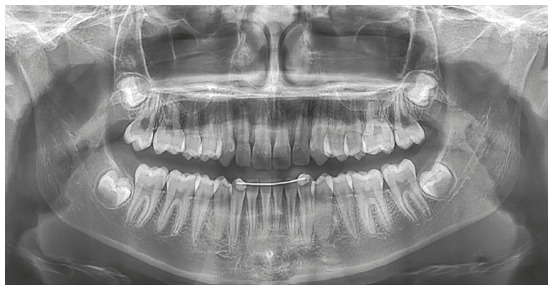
Case 2: Final panoramic radiograph.
